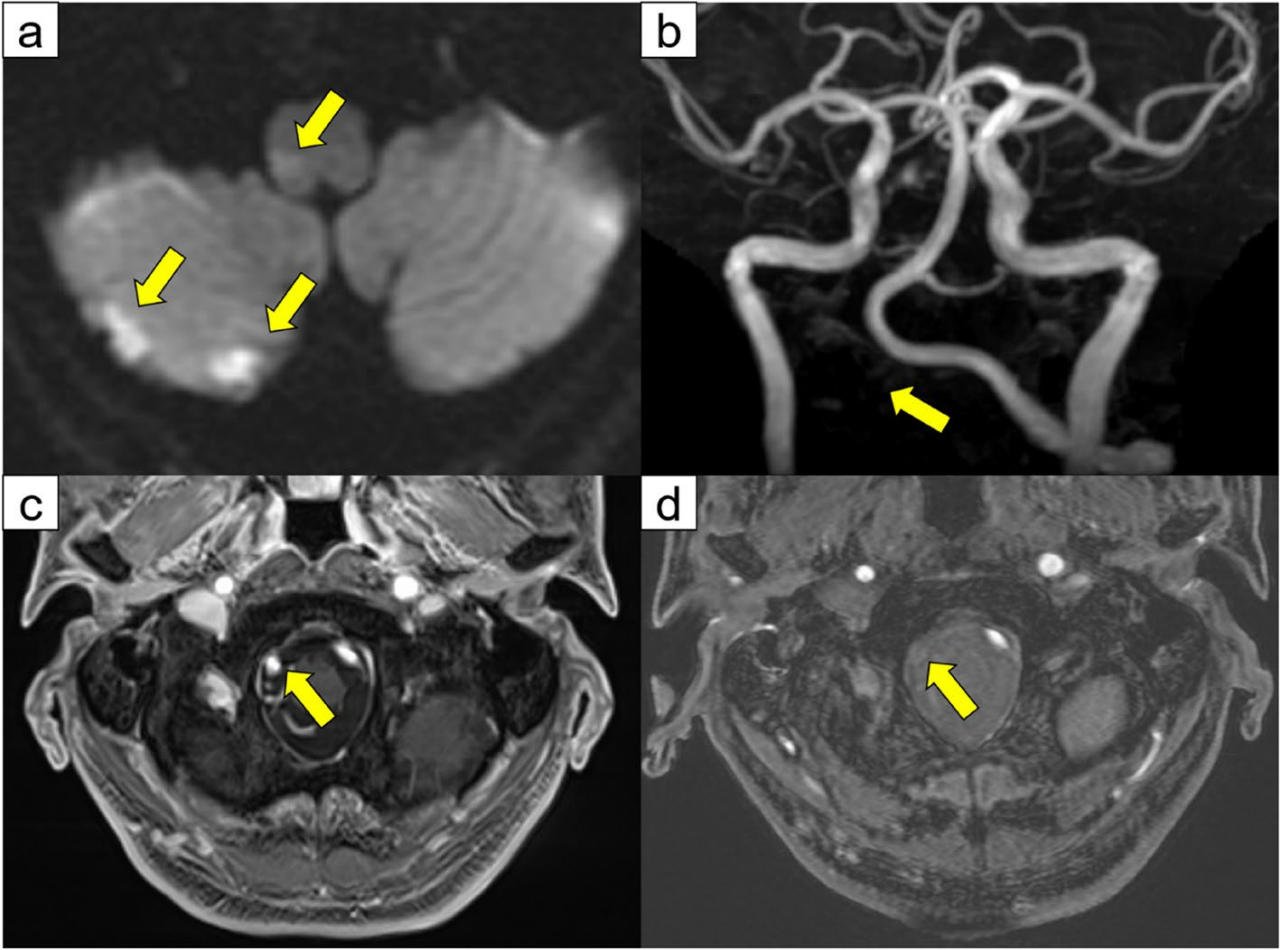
a Diffusion-weighted magnetic resonance image of the brain showing high-intensity signals in the medulla oblongata and the right cerebellum (yellow arrows). b Magnetic resonance angiography indicated occlusion of the right vertebral artery (yellow arrow). c The right vertebral artery can be seen on the preoperative head magnetic resonance image (yellow arrow). d Magnetic resonance imaging at the onset of cerebral infarction indicating a loss of signal in the right vertebral artery (yellow arrow)
